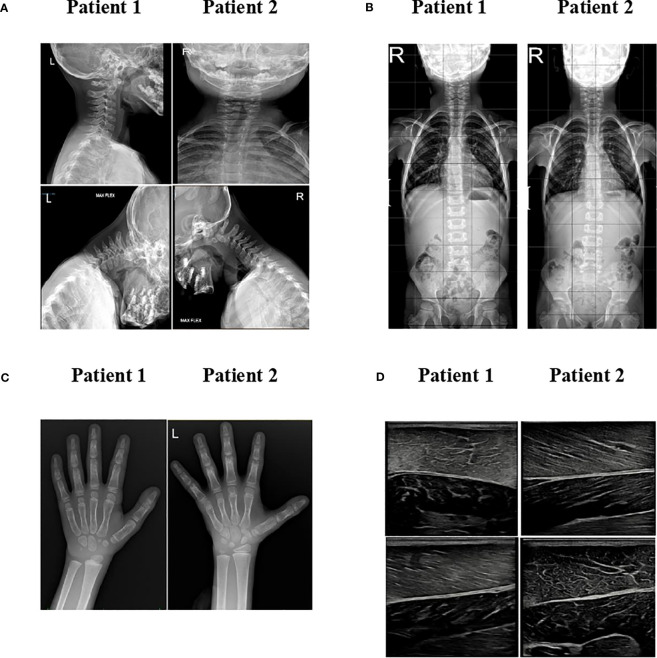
MRI imaging. T1-weighted MR imaging of patients 1 and 2 in comparison with a normal subject at different levels. Adipose tissue has a high signal intensity (brightness) on MRI T1-weighted images. When compared to normal subjects, the two patients have marked loss of subcutaneous fat, except for retro-orbital and bone marrow fat.
